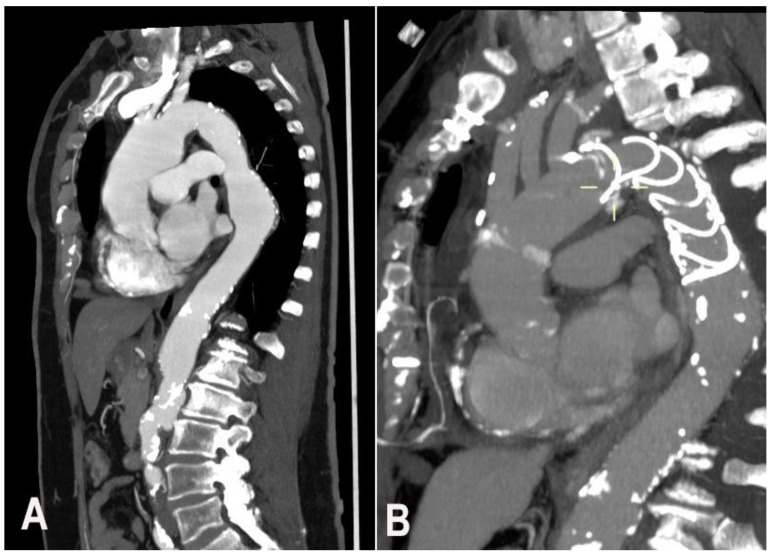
(A). Degenerative aneurism of the descending thoracic aorta, (B). Post FET CT scan.
